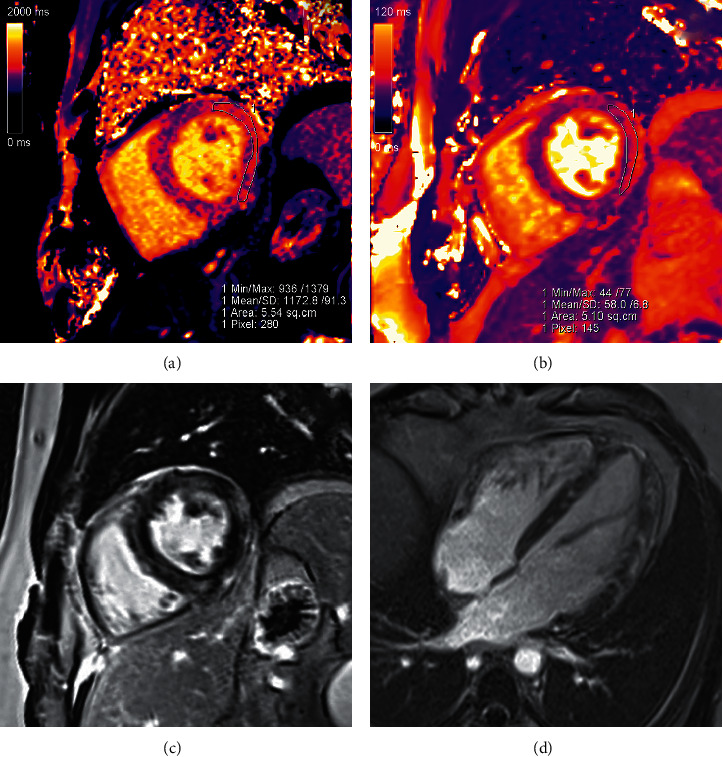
Cardiac MRI showed features consistent with myocarditis satisfying the updated Lake Louise criteria [4], visualized in these images. (a) Mid-short-axis native T1 mapping demonstrated elevated native myocardial T1 at 1173 ms (local lab normal reference: 950–1050 ms). (b) Mid-short-axis T2 mapping demonstrated elevated myocardial T2 at 58 ms (normal reference: 40–50 ms). (c) Mid-short-axis phase-sensitive inversion recovery late gadolinium enhancement image showed multiple areas of subepicardial enhancement in the lateral wall, as well as midmyocardial enhancement in the septum. (d) Four-chamber late gadolinium enhancement image demonstrated midmyocardial enhancement in the septum and subepicardial to midmyocardial enhancement in the lateral wall.
